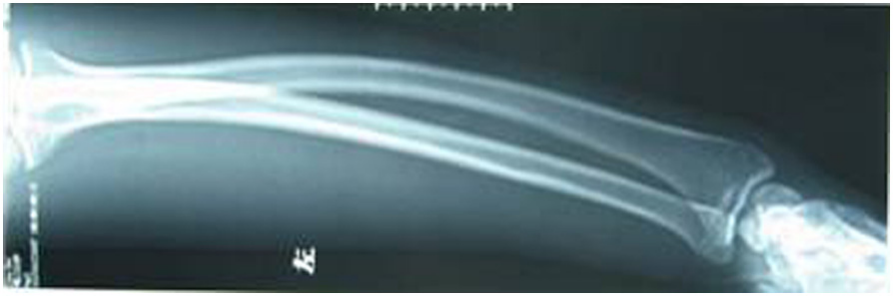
X-ray of both ulnar and radial plastic deformation.
